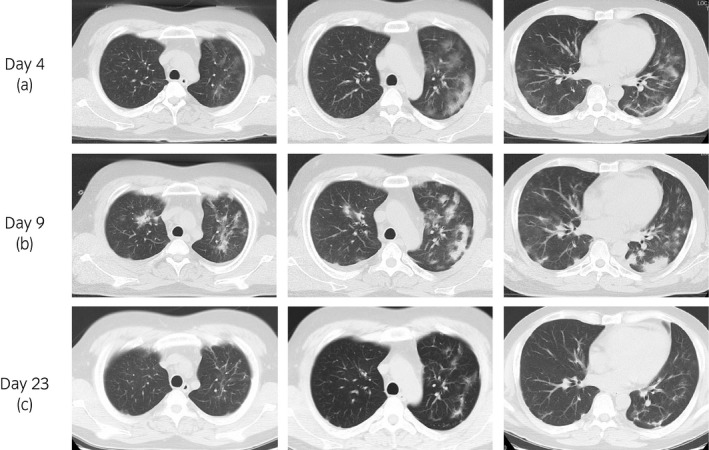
CT images of the patient. (a) On transfer to our hospital (day 4). CT reveals pulmonary ground‐glass shadow on both lungs. (b) Five days after transfer (day 9). CT shows pulmonary shadow getting worse. (c) Six days after low‐dose mPSL administration (day 23). Pulmonary shadow is almost disappeared on CT.
